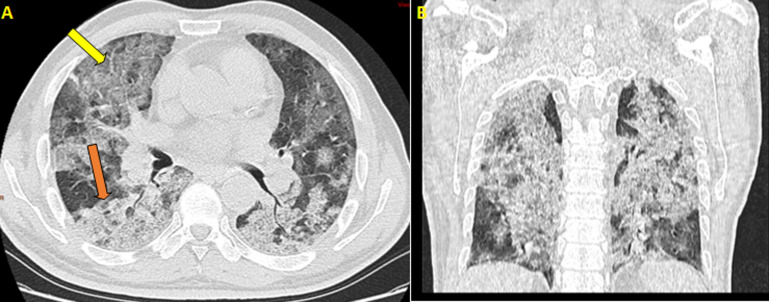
the first thoracic CT scan in an axial (A); and coronal (B) lung window showed ground-glass-opacities associated with crazy paving (yellow arrow) as well as the start of consolidation (orange arrow); the percentage of lung involvement is approximately 75%
